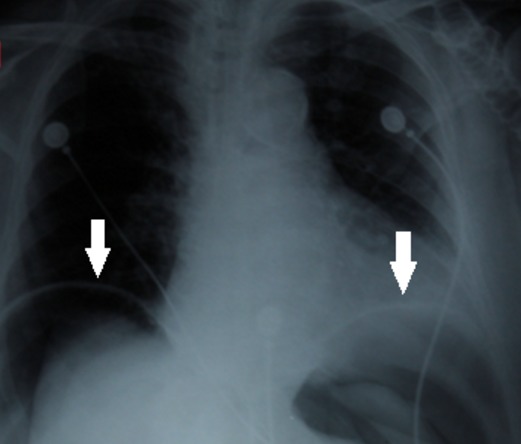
Chest X-ray at ICU admission after airway management and mechanical ventilation. It showed bilateral air collections in subphrenic areas (see arrows)
